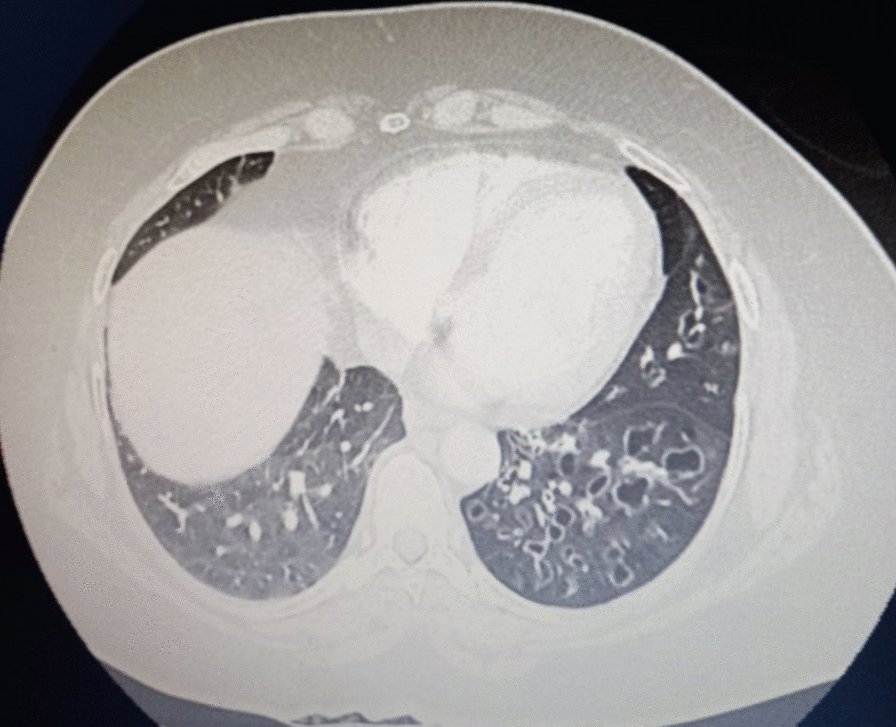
Chest computed tomography showing diffuse cystic bronchiectasis in the left lung
